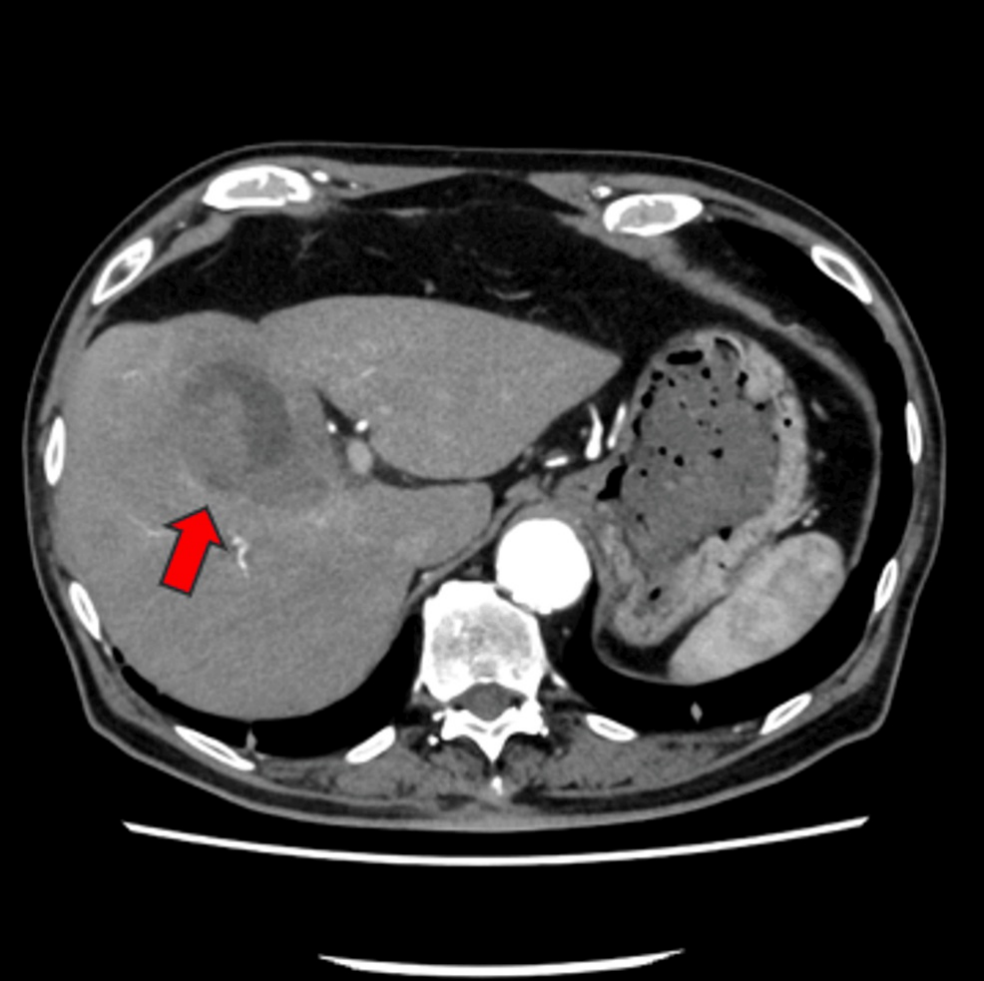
Contrast-enhanced CT on day 4 of hospitalization showed a swollen and edematous gallbladder (arrow).
